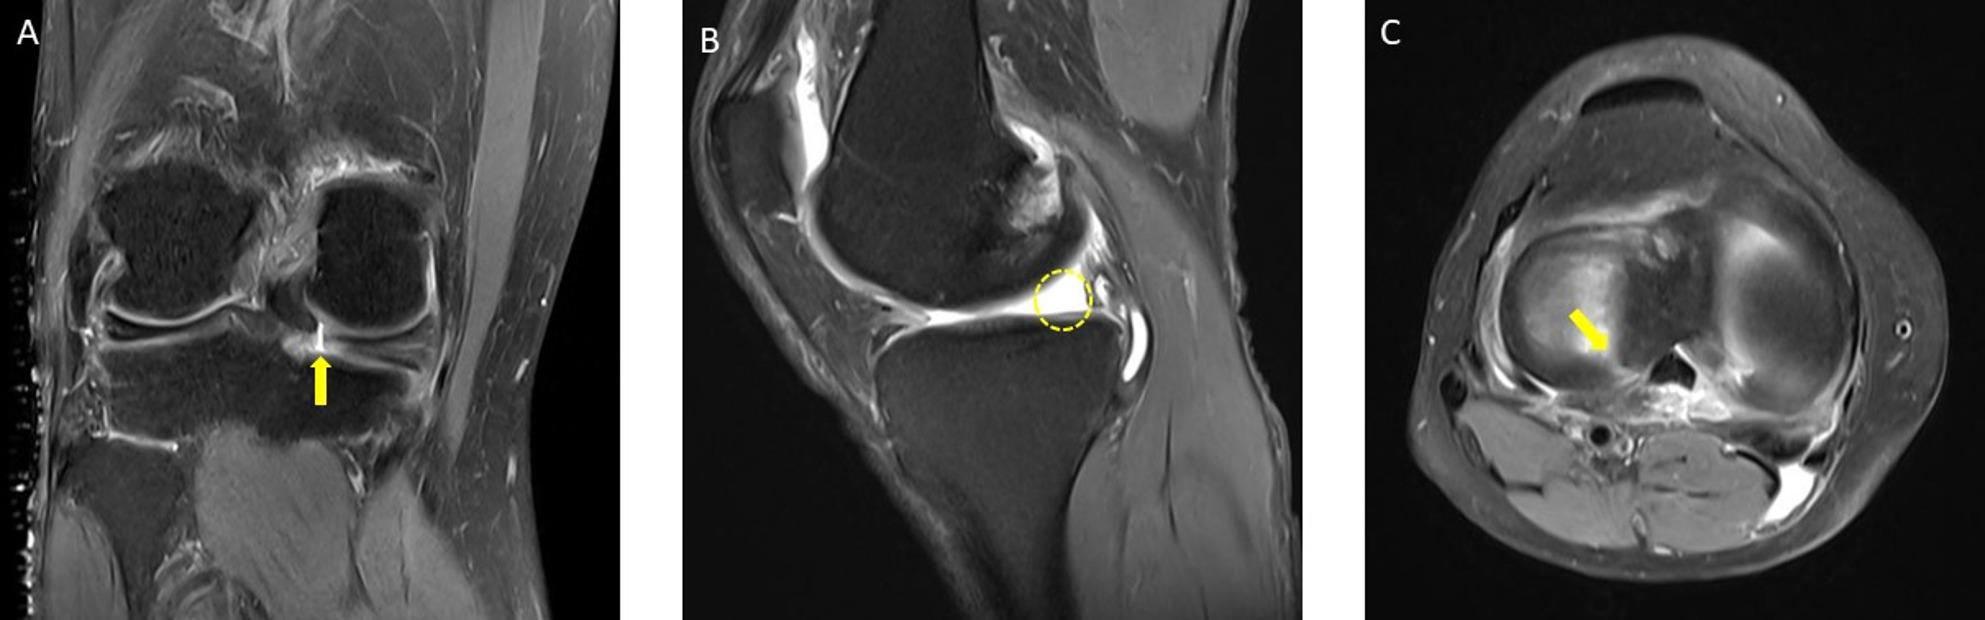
Representative images of magnetic resonance imaging (MRI) signs for meniscus posterior root tears (MPRTs). A, the cleft sign (indicated with an arrowhead, coronal image); B, the ghost sign (surrounded by a circle, sagittal image); C, the radial tear sign (indicated with an arrow, axial image)
